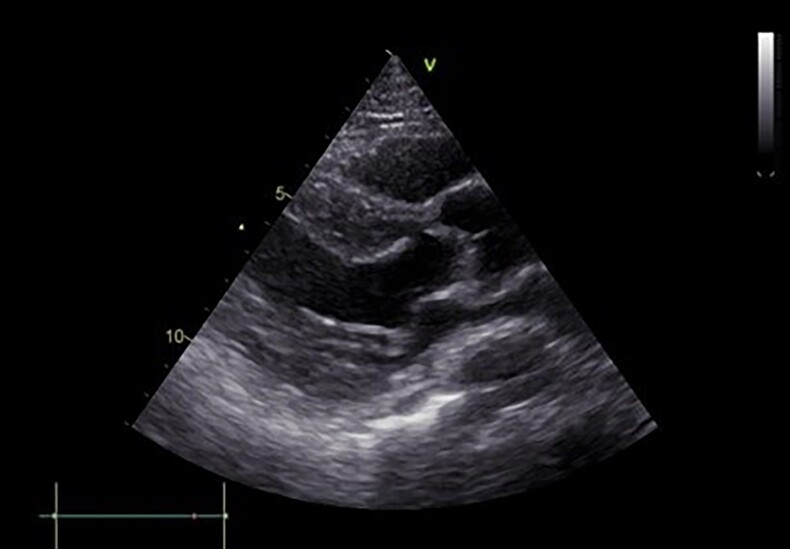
Parasternal long axis (PLAX) view of the transthoracic echocardiography, showing concentric left ventricular hypertrophy.
